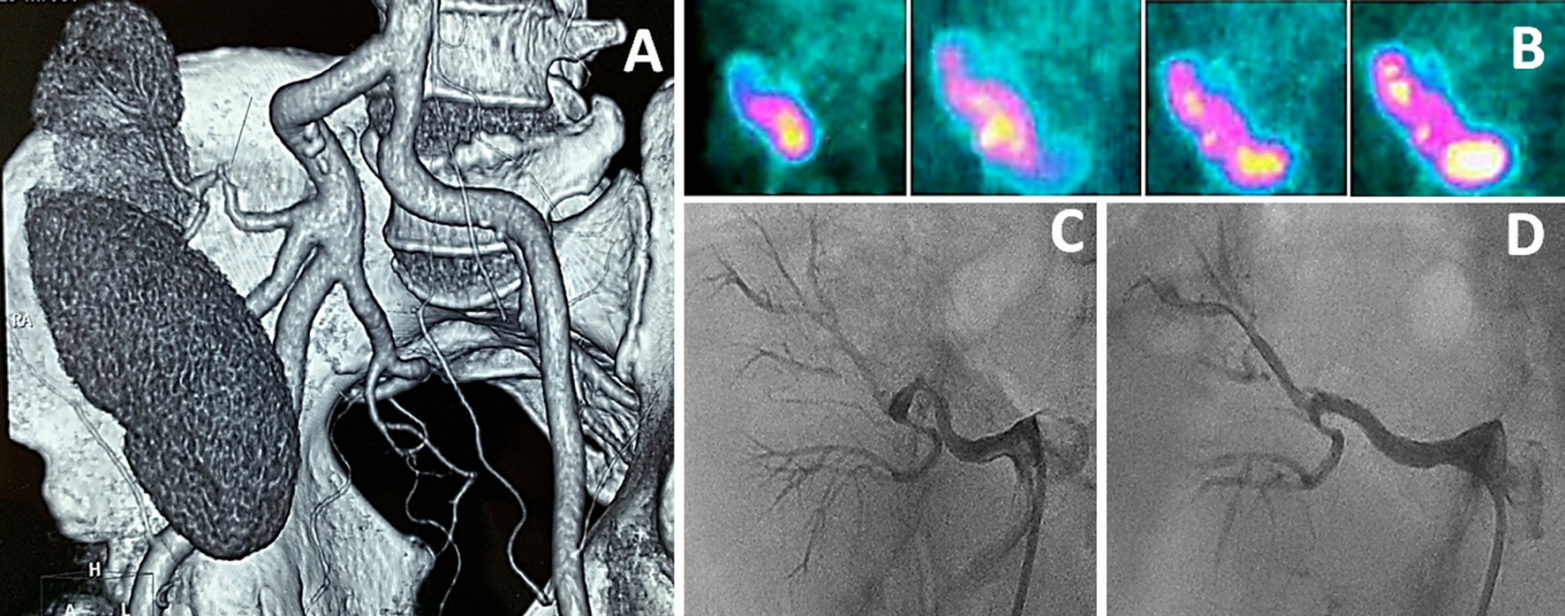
(A) Computed tomography (CT) image showed 80% stenosis of the transplanted renal artery (TRAS) of the posterosuperior kidney, while the renal artery of the anteroinferior kidney was normal; (B) A ^99m^Technitium diethylenetriamine-pentaacetate (DTPA) scan showed delayed radiotracer uptake by the cranially placed kidney, suggestive of TRAS; (C) A selective renal angiogram showed 80% stenosis of the transplanted main renal artery; (D) Following stenting, selective renal angiogram showed normal flow across the renal artery.
